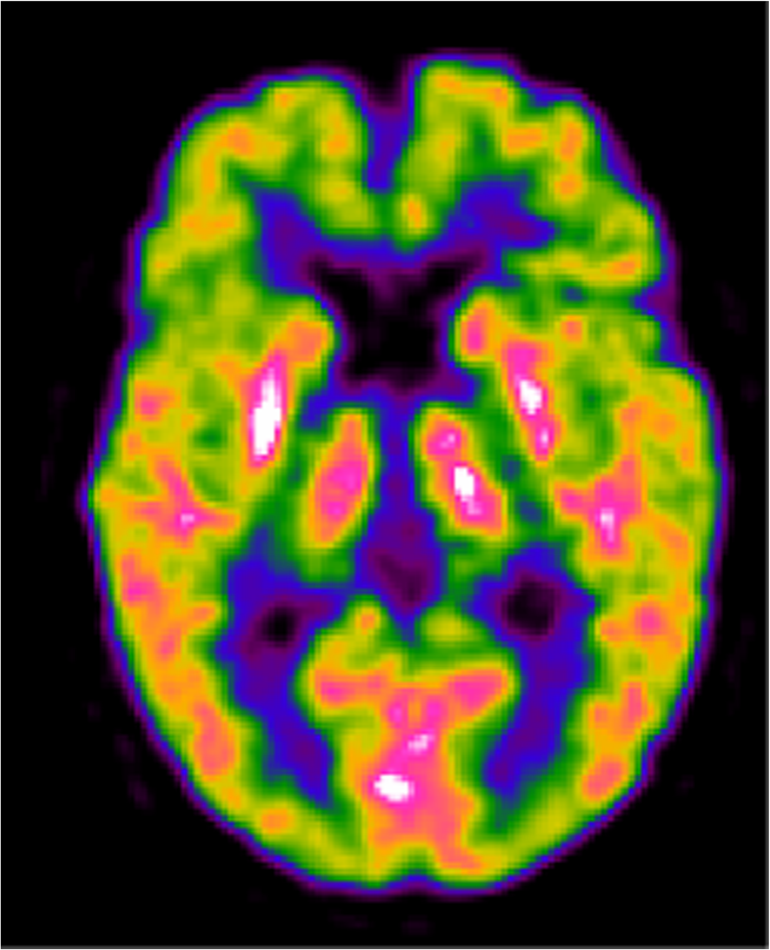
A 58-year-old patient with PSP and mild cognitive impairment (non-amnesic multiple domain MCI; MMSE score 26/30). The FDG PET image shows moderate hypometabolism of the bilateral frontal cortex, including the medial frontal gyri, and hypometabolism of the caudate nuclei mainly in the right hemisphere, and in the right thalamus
